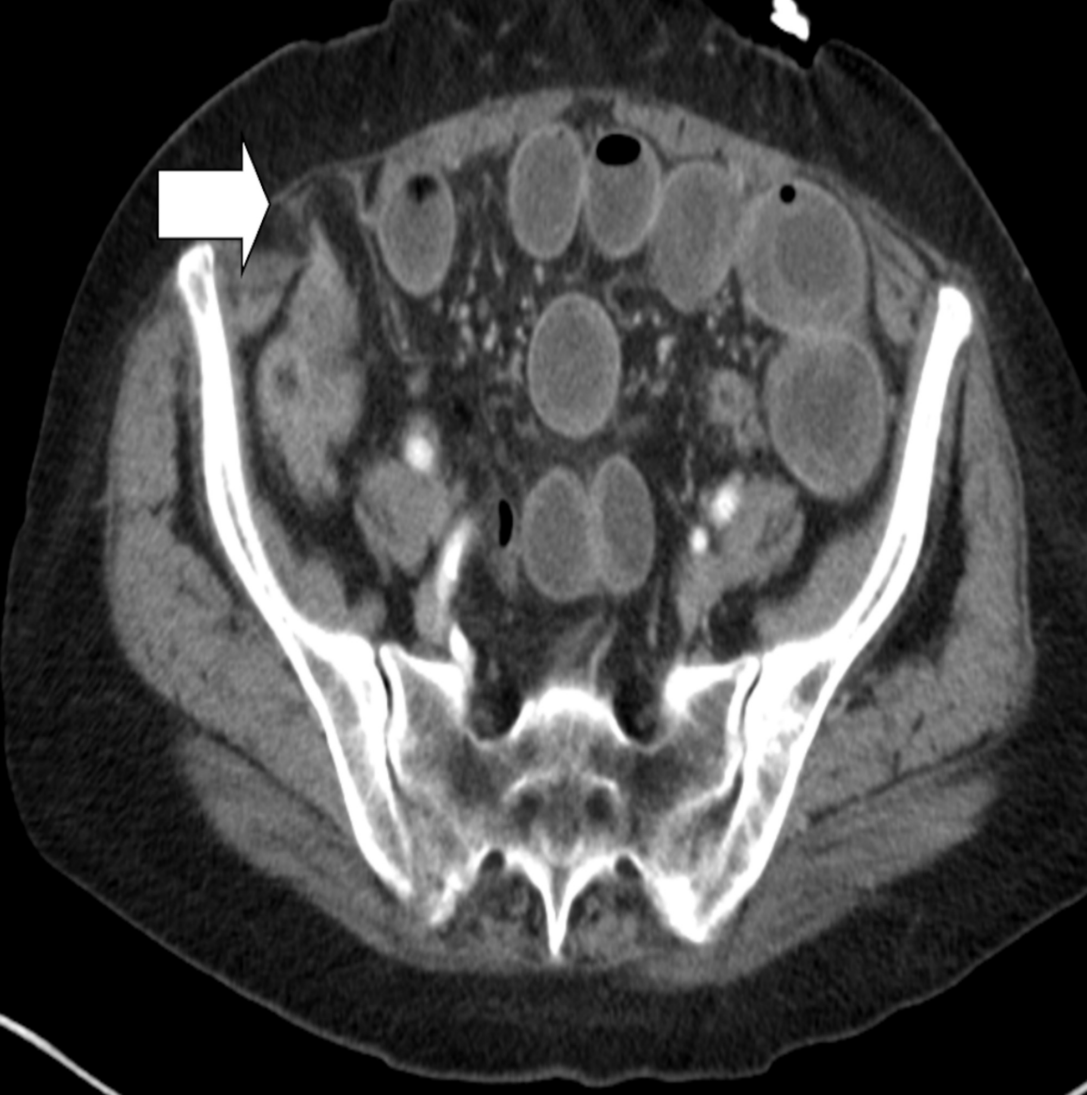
Computed tomography (CT) scan showing a Spigelian hernia defect as the transition point for small bowel obstruction (white arrow)
